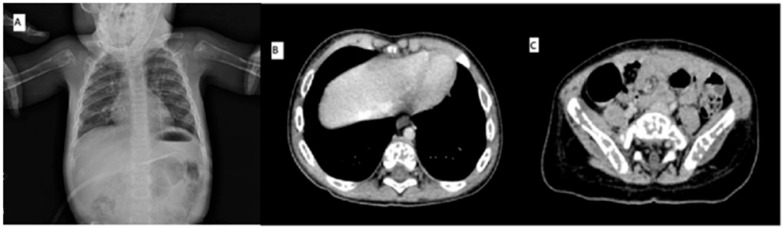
Skeletal X-ray (A) and total-body CT scan (B,C) showing widespread osteolytic areas. (A) Skeletal X-ray showing diffuse osteolytic lesions involving ribs and long bones; (B) total-body CT scan with contrast detecting multiple osteolytic areas in the axial and appendicular skeleton, particularly affecting thoracic vertebrae and pelvic bones.
